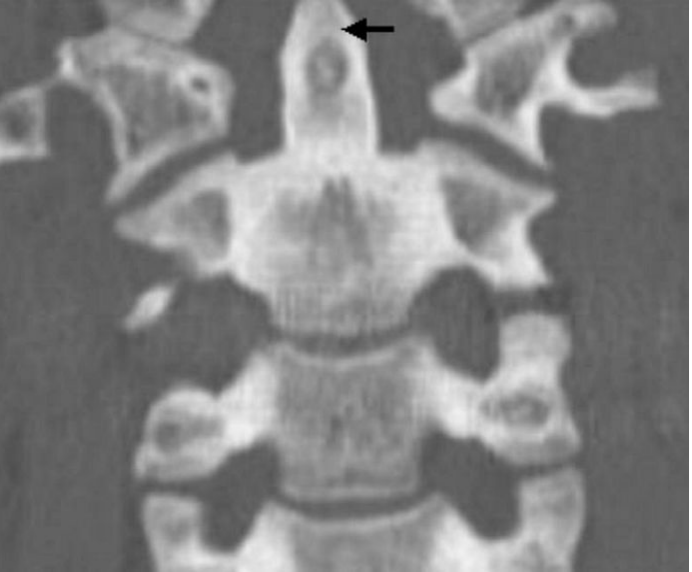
(patient 2). Coronal reformatted multiplanar computed tomography of the cervical spine, demonstrating the increased density of the transverse processes and the unduly long odontoid process.
